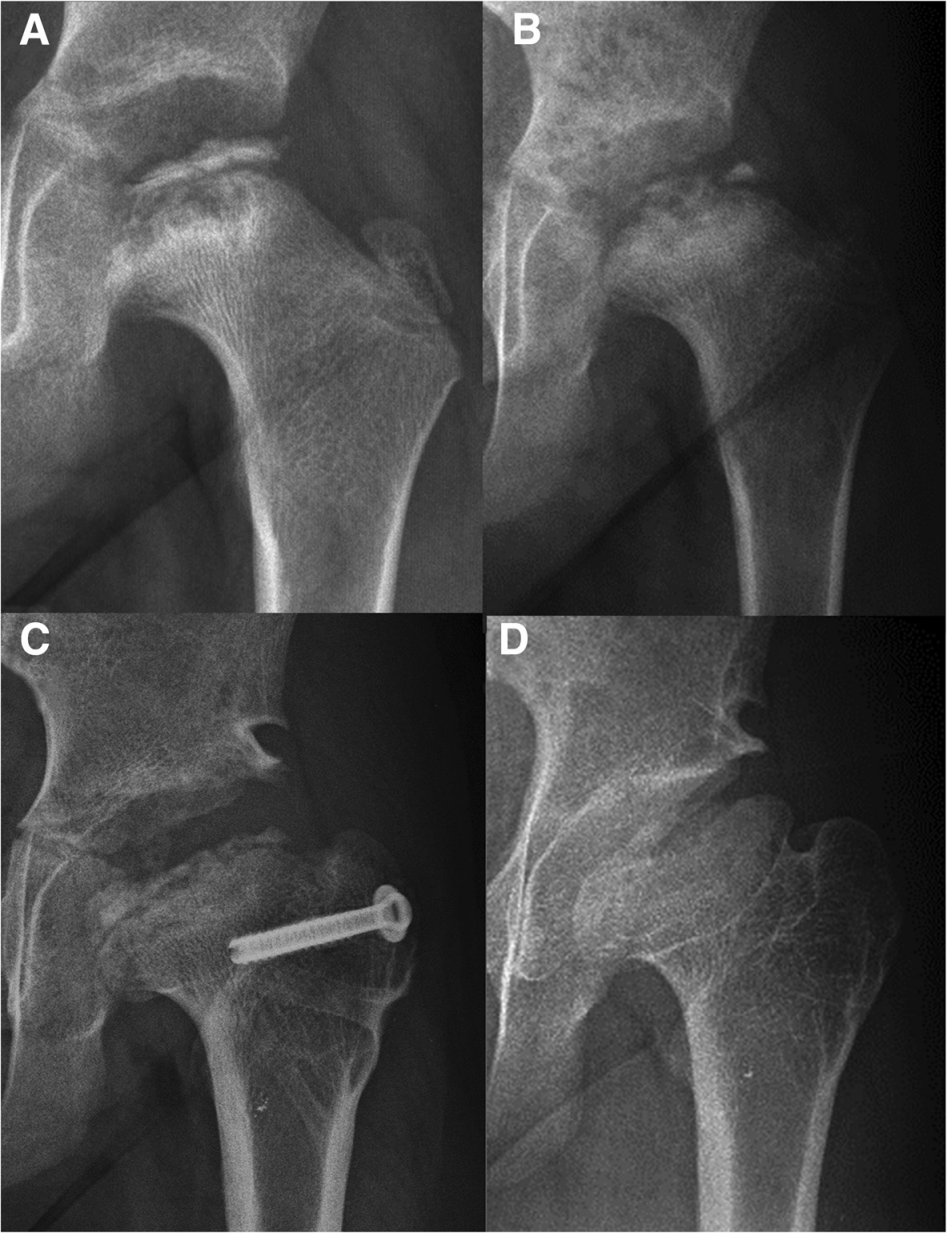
Sequential radiographic change of AVN in an MATN3-MED patient. A: Sclerotic femoral head and metaphyseal cyst at 9 years of age. B: Resorption phase at 10 years. C: Resossification phase at 12 years. D: Late reossification phase at age 15 years.
